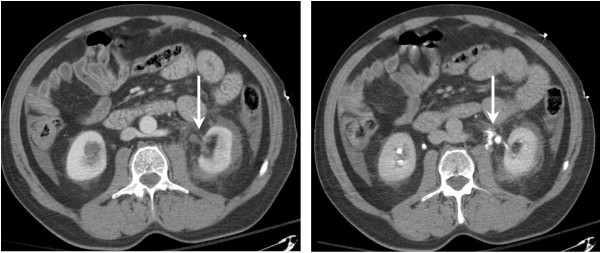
69 year old male. Contrast enhanced (left) and delayed (right) CT scans demonstrating low density fluid around the left kidney and ureter, and contrast extravasation from the proximal left ureter respectively.
